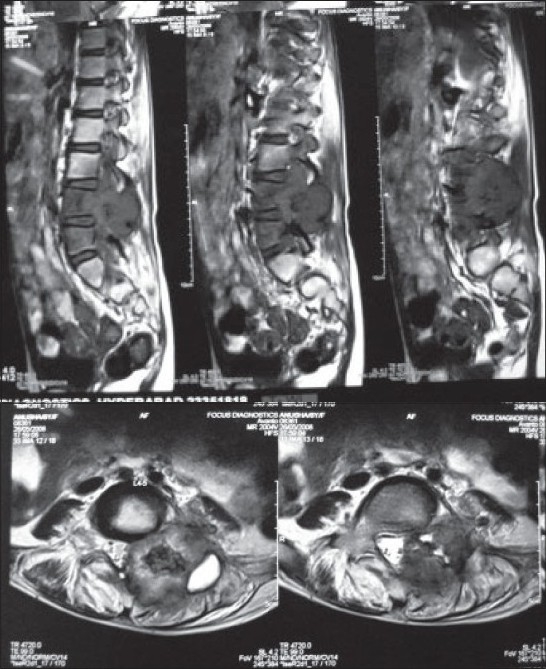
Magnetic resonance imaging images show the lesion in posterior elements with edema extending into the spinal canal, L4, L5 vertebral bodies and intervening disc
